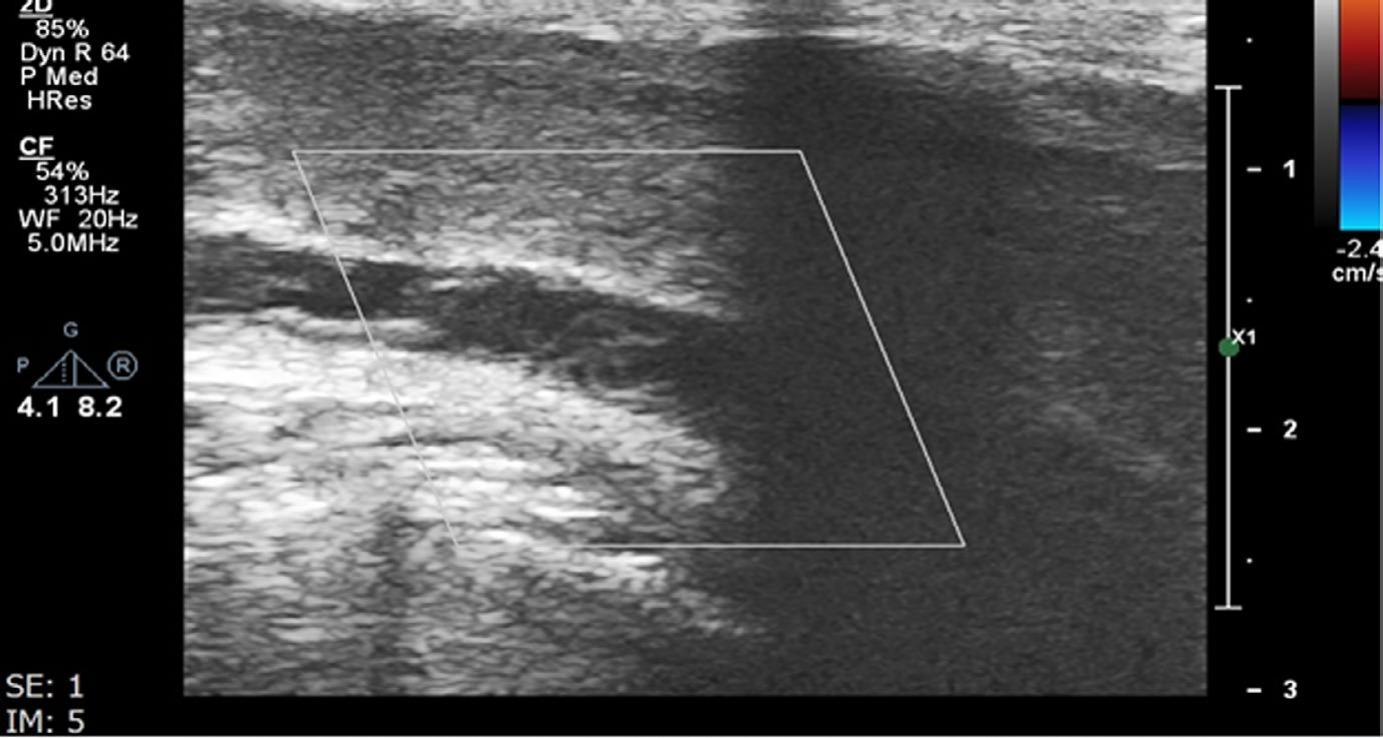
Ultrasound evaluation of the deep dorsal penile vein shows no flow in the vein. Also the vein is dilated and contains echogenic thrombosis from the middle part of the penis extending to the root of the penis at posterior of the pubic symphysis. The thrombosis is not extended to the superior of the urogenital diaphragm. Above findings are in favor of subacute thrombosis of the deep dorsal penile vein
